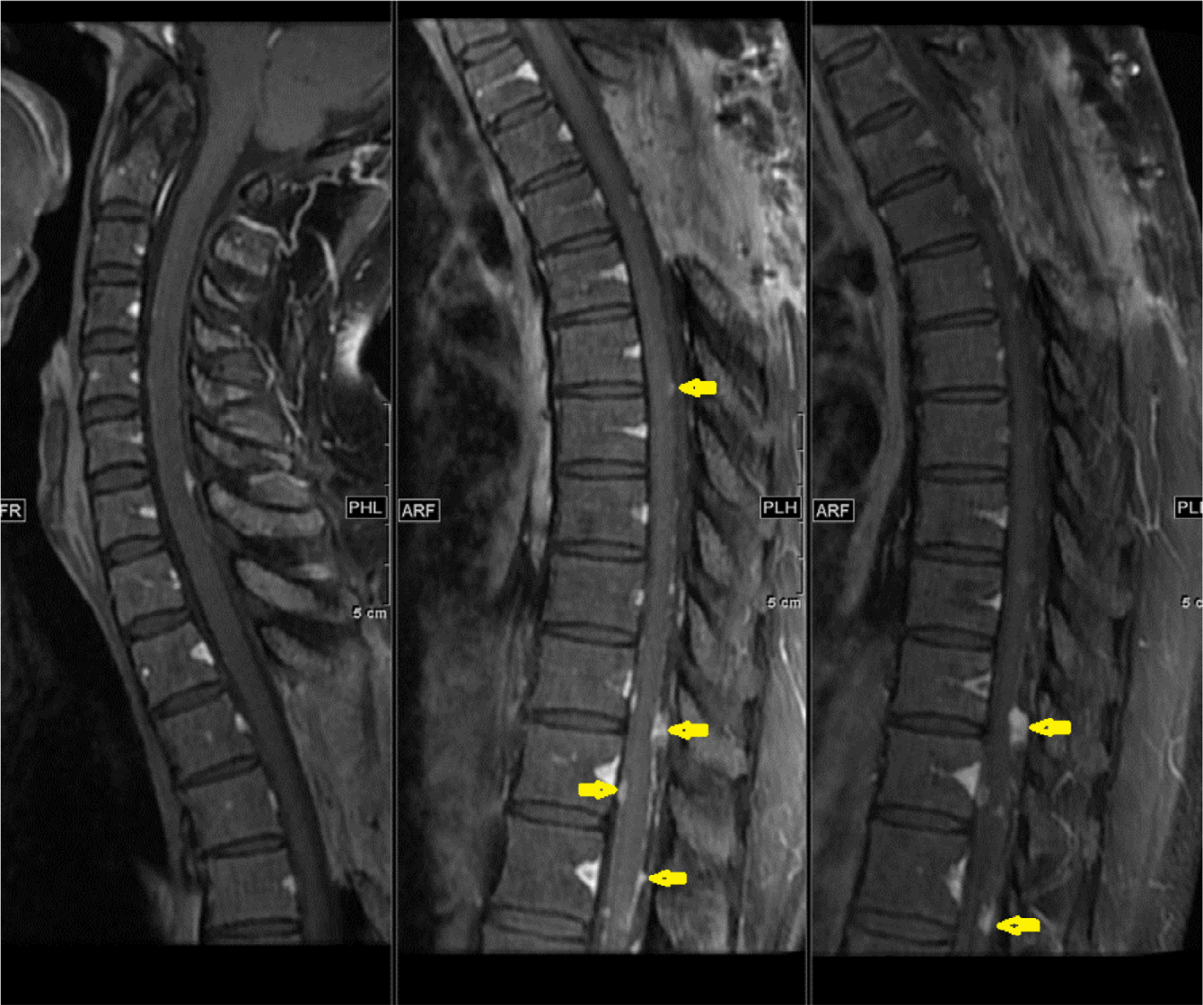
Sagittal MRI images of cervical and thoracic spine 48-months post-surgery show post-surgical changes in the upper thoracic spine following resection. Enlarging and new enhancing nodules consistent with CSF seeding (arrows)
